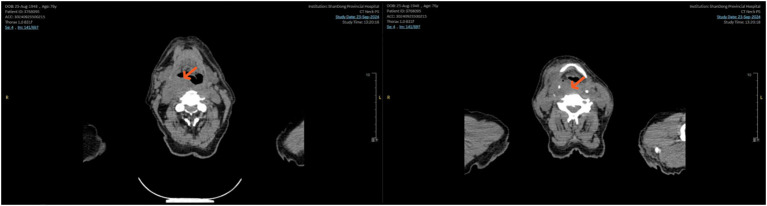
Representative CT scan of Patient 1 with deep neck space infection. Axial contrast-enhanced CT imaging demonstrates soft-tissue swelling and heterogeneous low-density areas involving the retropharyngeal space, right parapharyngeal space, and submandibular region. The irregular contours and fluid-attenuation areas are consistent with abscess formation within multiple deep cervical compartments.
